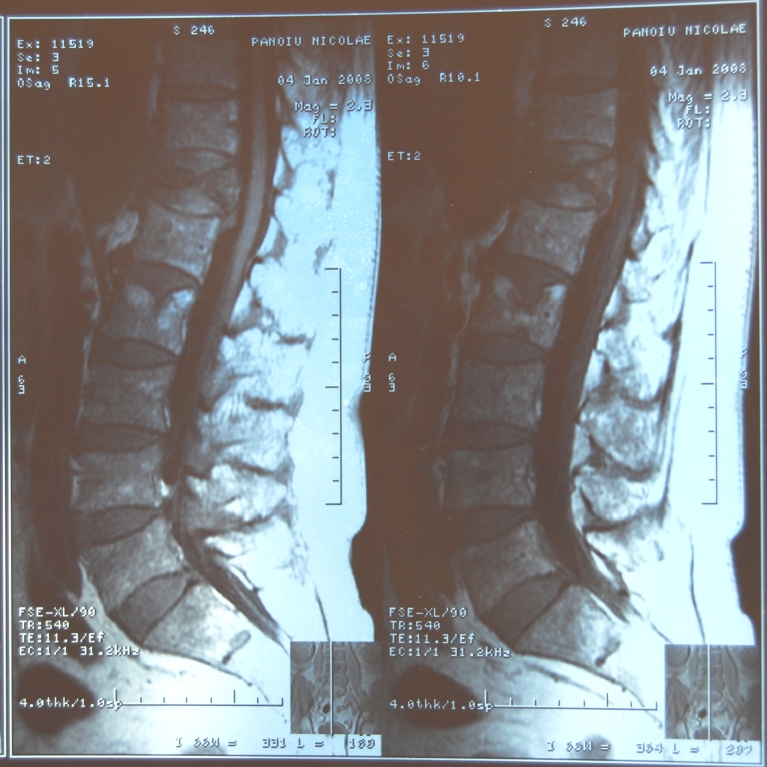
Spinal MRI scan – compression fracture of T12, L1, L2 with collapsed plateau – P.N. case
